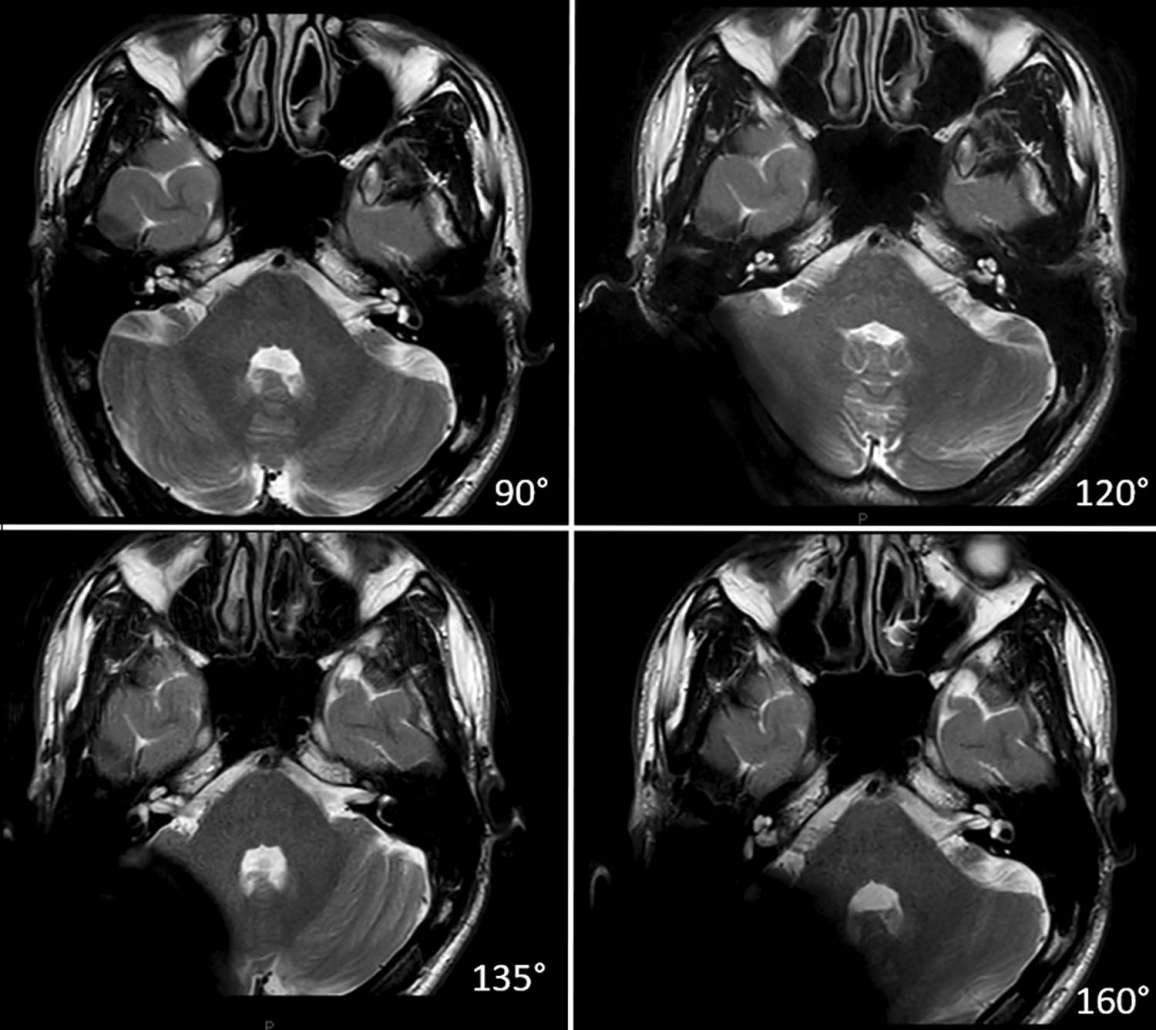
Ipsilateral inner ear and internal auditory canal MRI visibility according to each CI position on 2D axial T2w sequences
